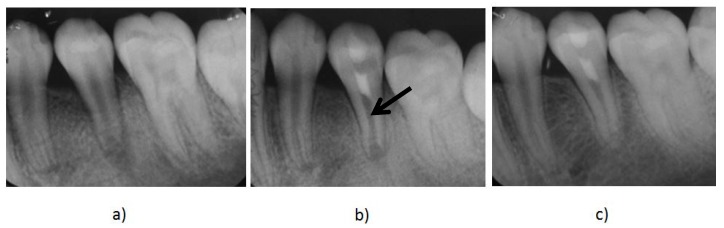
(a) A preoperative radiograph of an immature mandibular second premolar with pulp necrosis and apical periodontitis. (b) Immediate post-treatment radiograph of the tooth after the treatment with regenerative endodontic procedures (REPs). The mineral trioxide aggregate (MTA) is the radiopaque intracanal barrier (black arrow) that was placed over the induced blood clot that acted as a scaffold to the introduced stem cells promoting further root development. (c) A follow up radiograph taken 12 months after treatment shows further root maturation and evidence of thickening of the canal walls and apical closure.
